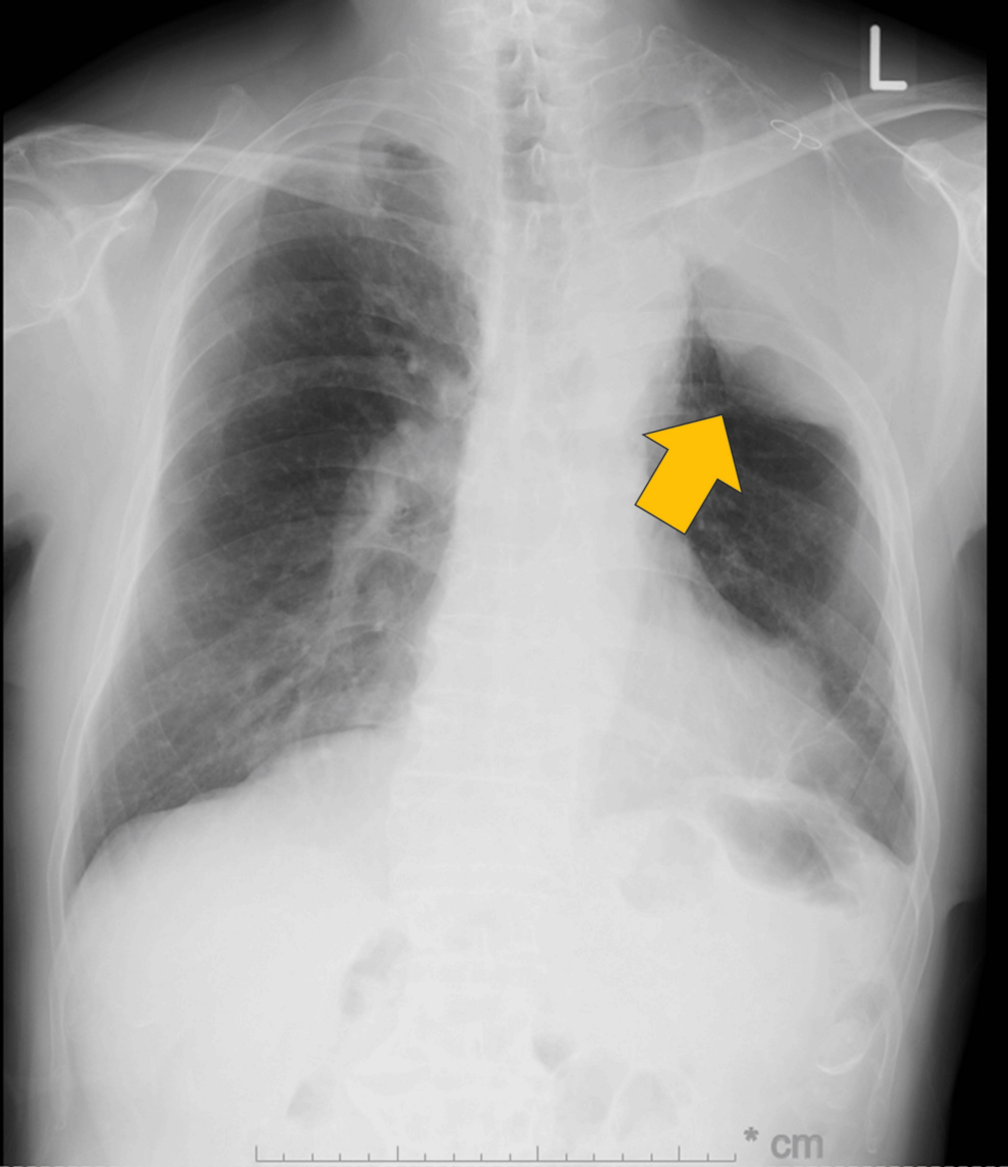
Chest X-ray findings Extraperiosteal paraffin plombage space was observed in the left upper lung field (arrow).
